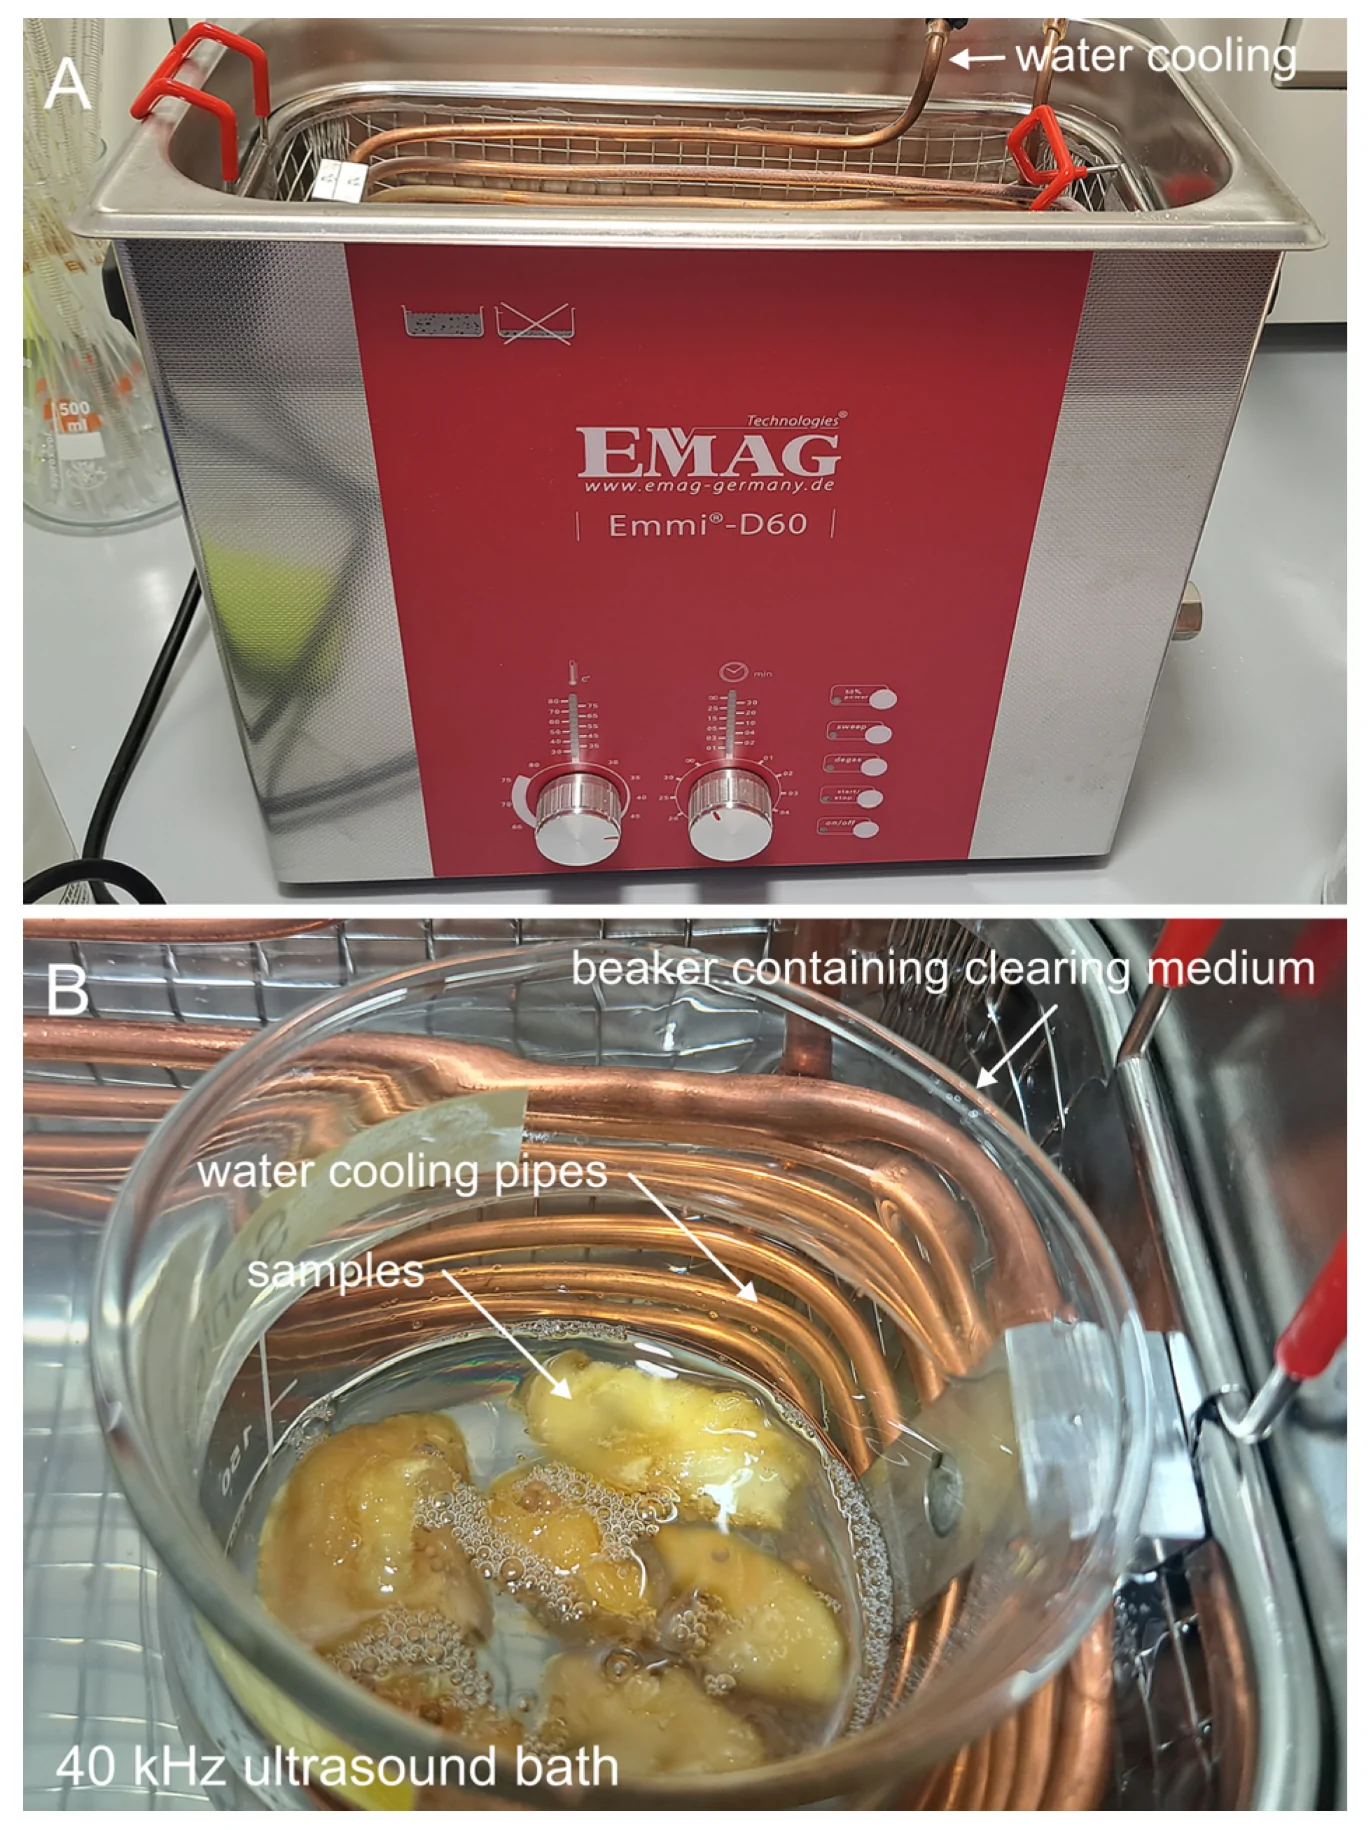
Experimental setup for 40 kHz ultrasound-assisted tissue clearing. (A) The standard 40 kHz ultrasound cleaning bath (Emmi-D60, EMAG) used for the experiments. (B) View of the water tank: A beaker containing the tissue samples and clearing medium (DBE) is immersed in the water bath. A custom-made spiral copper tube circulates cold tap water to remove the heat generated by the transmitted ultrasound energy, maintaining the temperature at approx. 20 °C.
